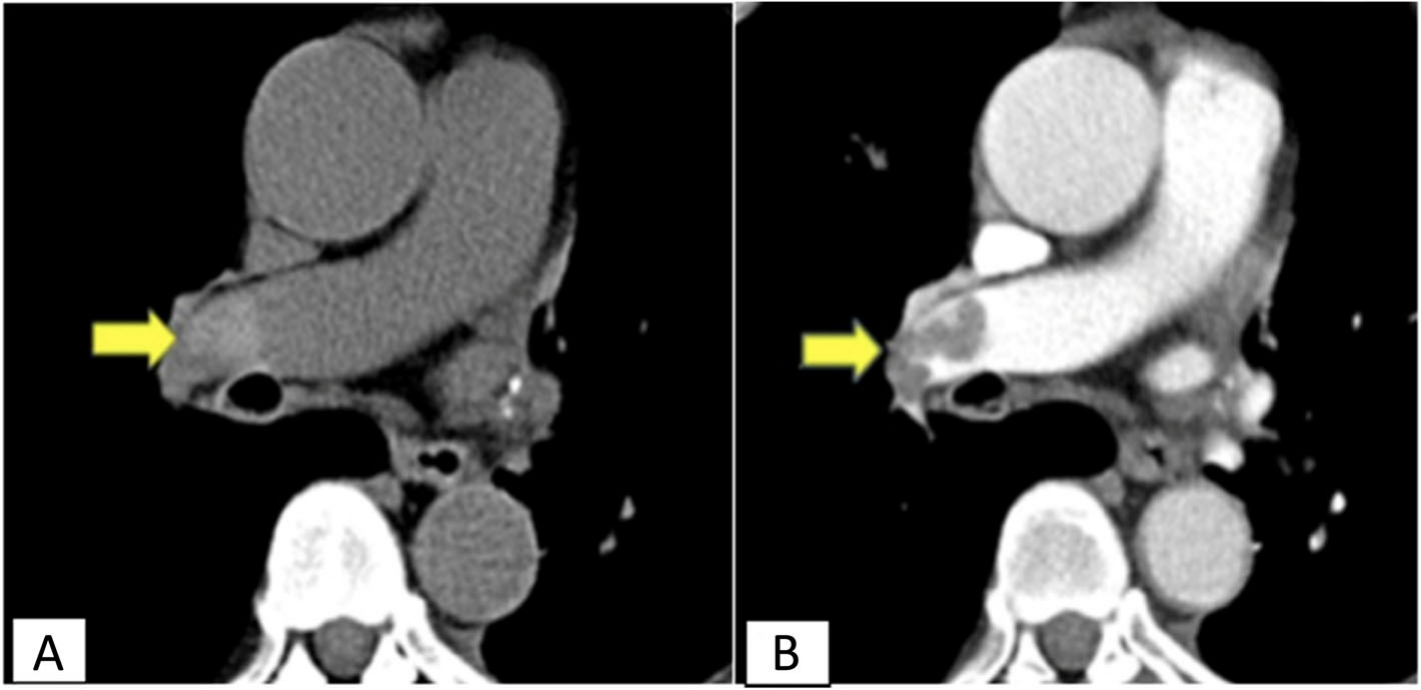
a Axial non contrast CT image. b Axial CTPA image shows a case of acute pulmonary embolism with hyperdense thrombus seen at right main pulmonary artery (arrows in a) with corresponding filling defect at right main pulmonary artery (arrows in b)
